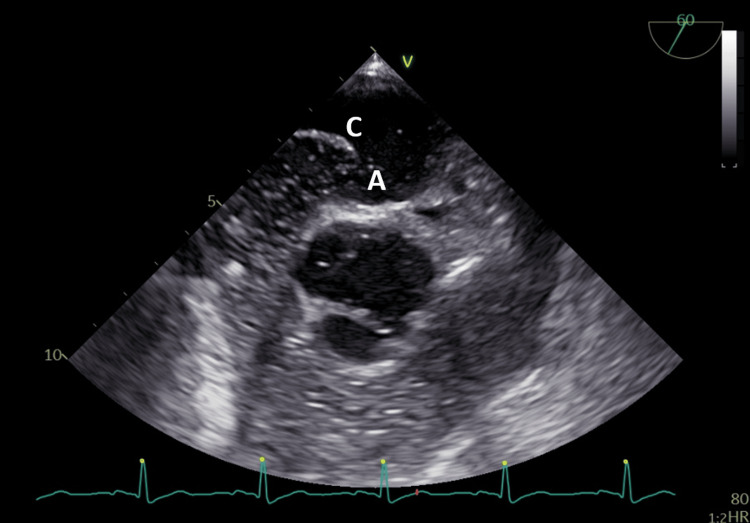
Transesophageal echocardiogram with bubble test showing patent foramen ovale with shunt (A) and atrial septal aneurysm (C).
